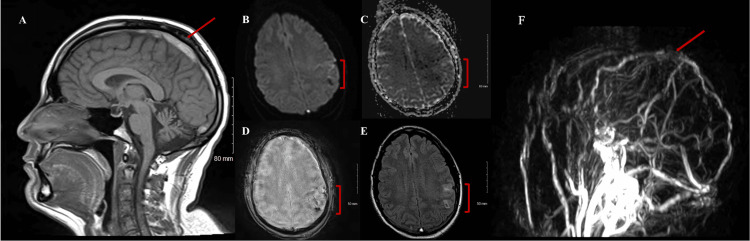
MRI and MRV of the Brain without Contrast (A) T1 Sagittal MRI of the brain showing thrombosis of the superior sagittal sinus. (B) Diffusion-weighted imaging and (C) apparent diffusion coefficient sequences of the brain MRI showing no restricted-diffusion. (D) T2 star and (E) FLAIR sequences of the brain MRI showing the intraparenchymal hemorrhages. (F) MRV showing thrombosis in the superior sagittal sinus. MRI: magnetic resonance imaging; MRV: magnetic resonance venography; FLAIR: fluid attenuated inversion recovery
